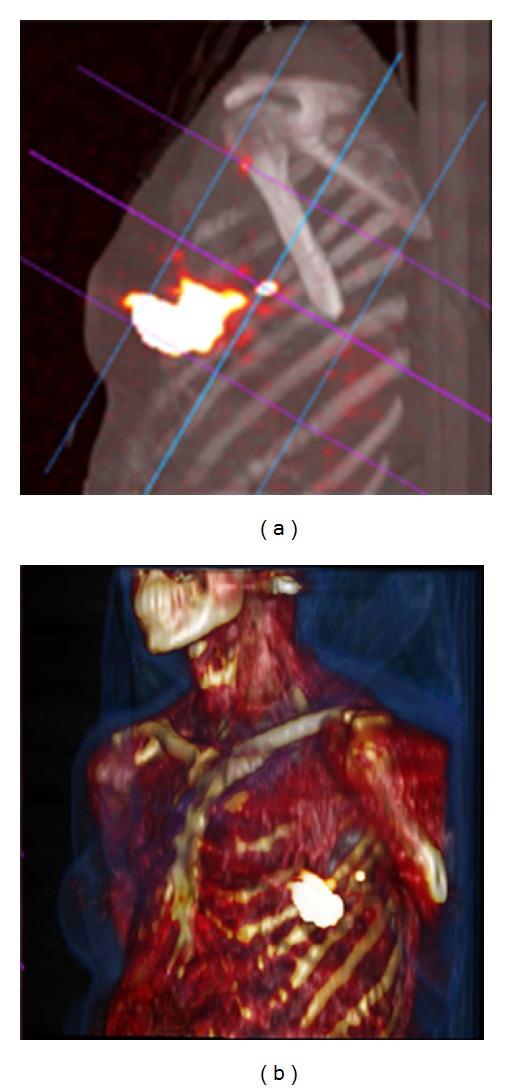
Fused SPECT/CT (a) displayed with maximum intensity projection (MIP) showing a sentinel node in the left axilla. SPECT/CT with volume rendering for 3D display (b) adds an excellent overview for surgical planning.
